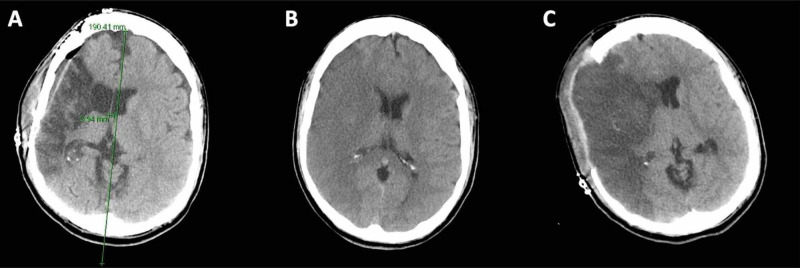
(A) CT reveals a 3.94 mm midline shift postoperatively with a clear right MCA infarct. (B) CT reveals a region of hypodensity indicative of malignant right-sided MCA infarct. (C) Post-decompressive surgery CT scan showing a large malignant MCA infarct with less mass effect. CT: Computed tomography MCA: Middle cerebral artery
